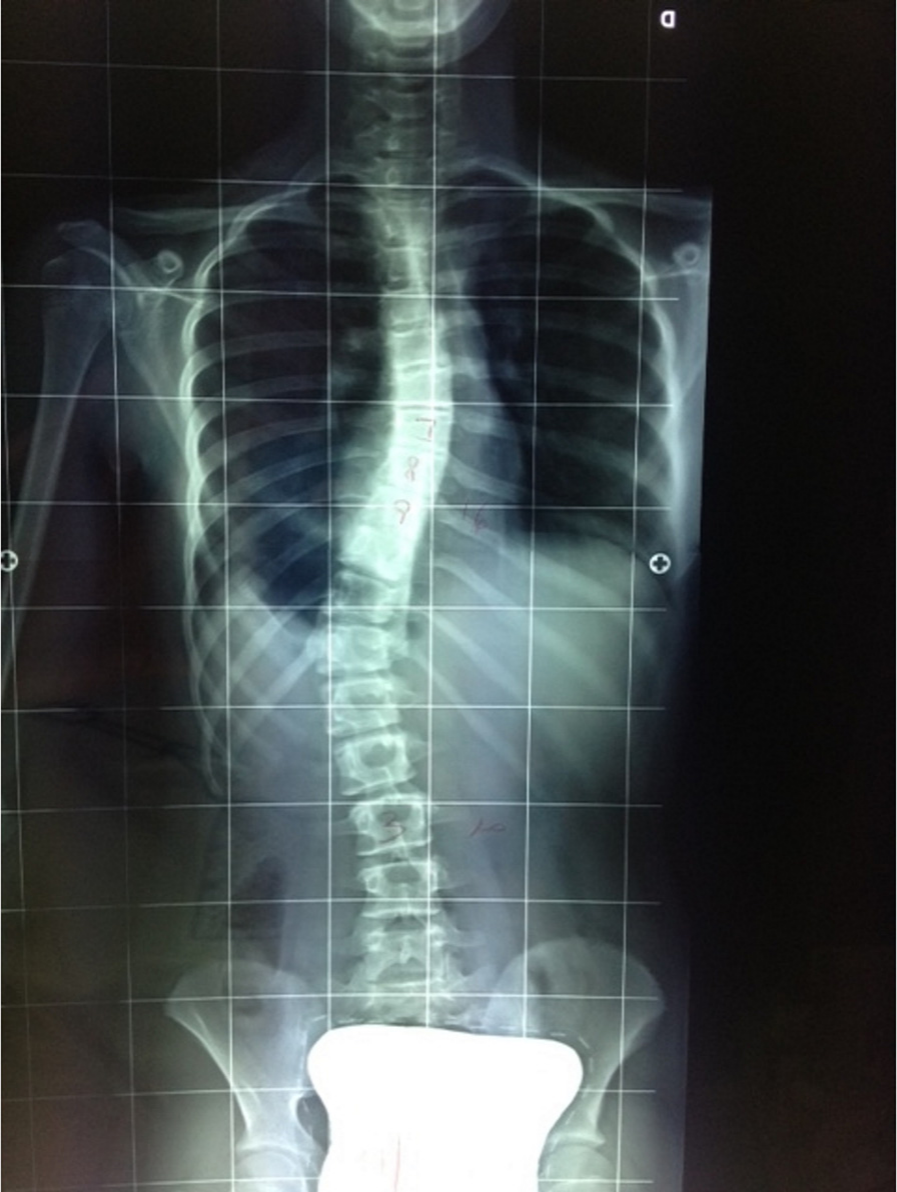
Patient 1. Posterior view Postero-Anterior Spine X-ray while the patient maintains the active slf-correction learned during the exercise session.
